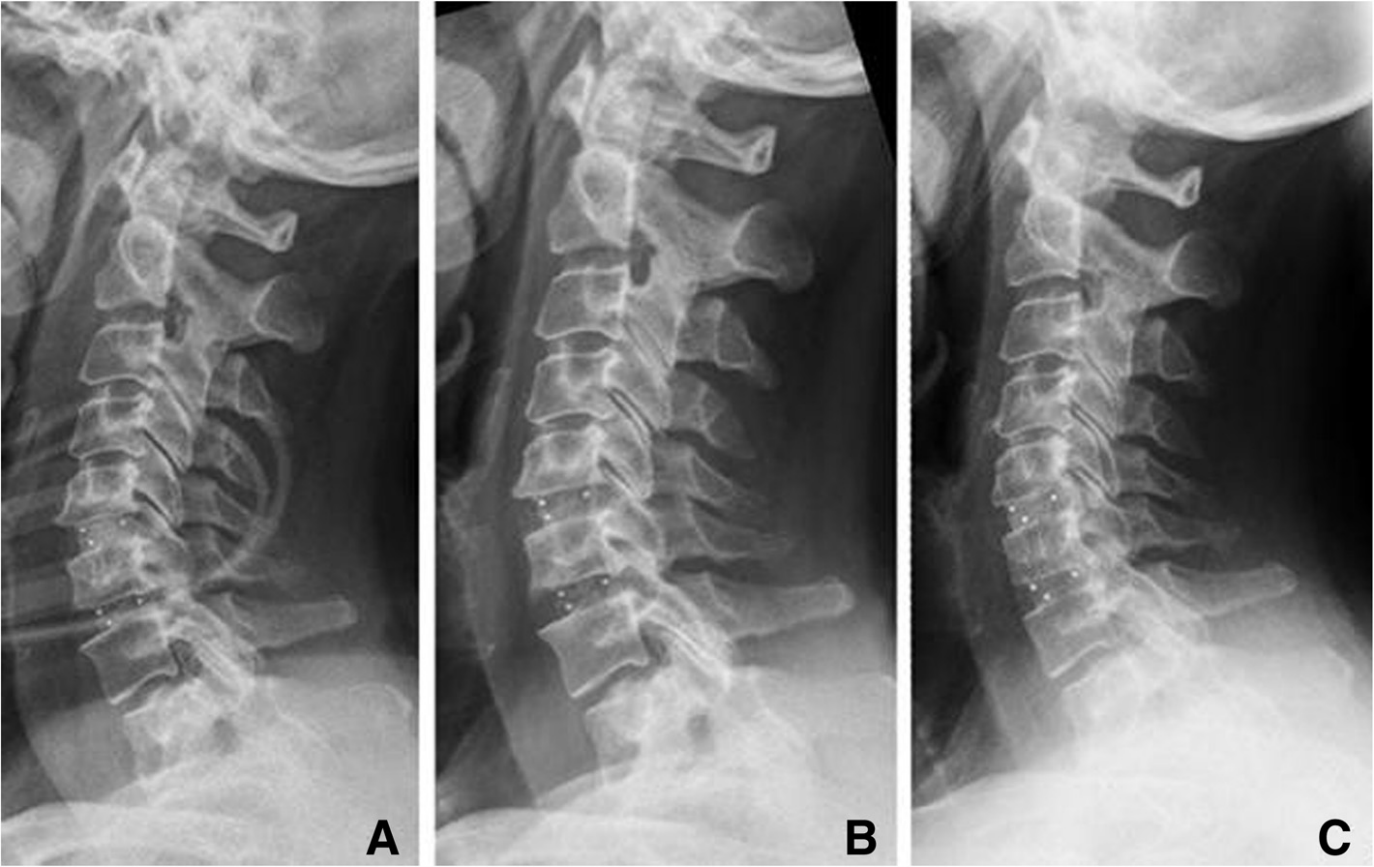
Cervical spine (female, 66 years) with spinal stenosis in C5/6 and C6/7 and myelopathy treated by cervical cages in C5/6 and C6/7 – X-rays taken A immediately postoperatively, B 1 year after surgery without cage subsidence, and C 3 years postoperatively with osseointegration without subsidence.
